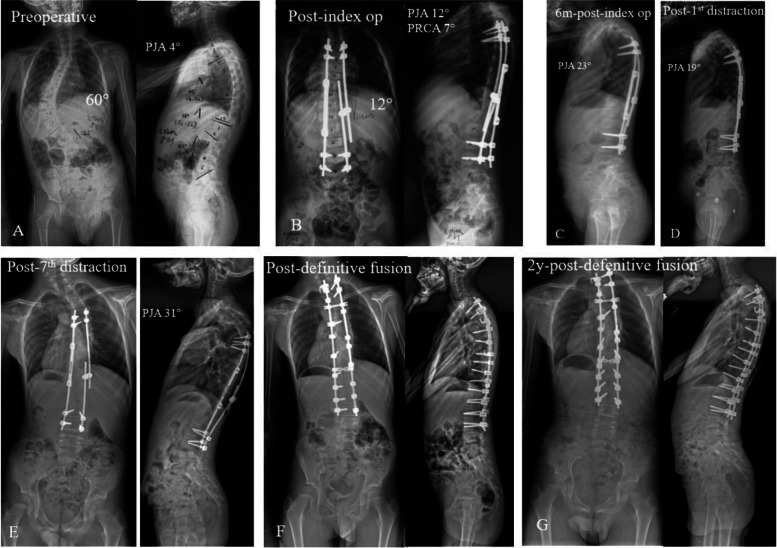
This 5-year-old boy was diagnosed with idiopathic scoliosis, showing a 60° Cobb angle and 4° PJA before surgery (A). After the index surgery, the radiographs showed a 12° PJA and a 7° PRCA (B). At 6-month follow-up, the PJA increased to 23°(C). After the first lengthening surgery, the PJA was 19° (D). After completion of 7 years of follow-up (7 lengthening), the PJA increased to 31° (E). This patient underwent definitive fusion at the age of 13 years old and the PJK was corrected (F)
